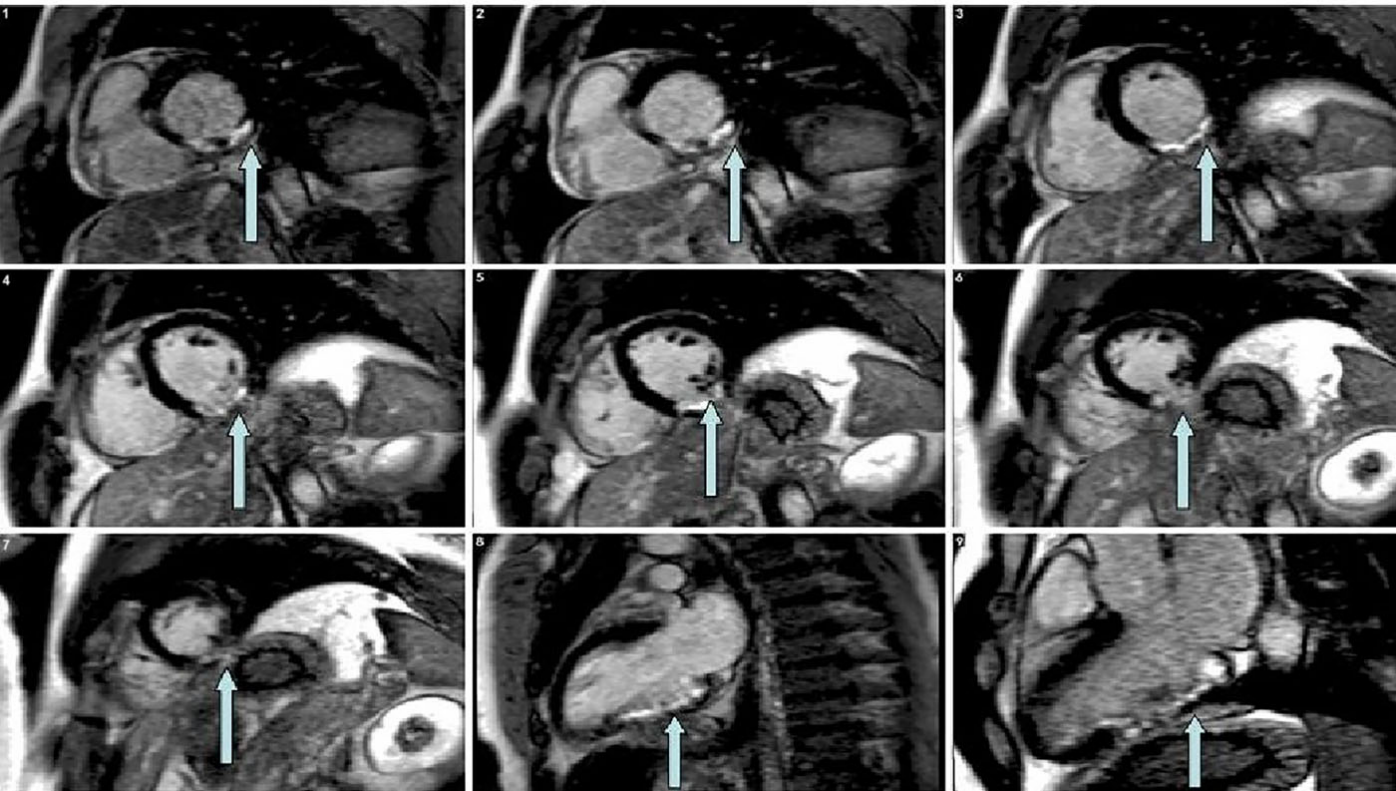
Cardiac functional MRI, with multiple images from different angles and with arrows pointing to scarring amounting to 16.3% of the left ventricle.
